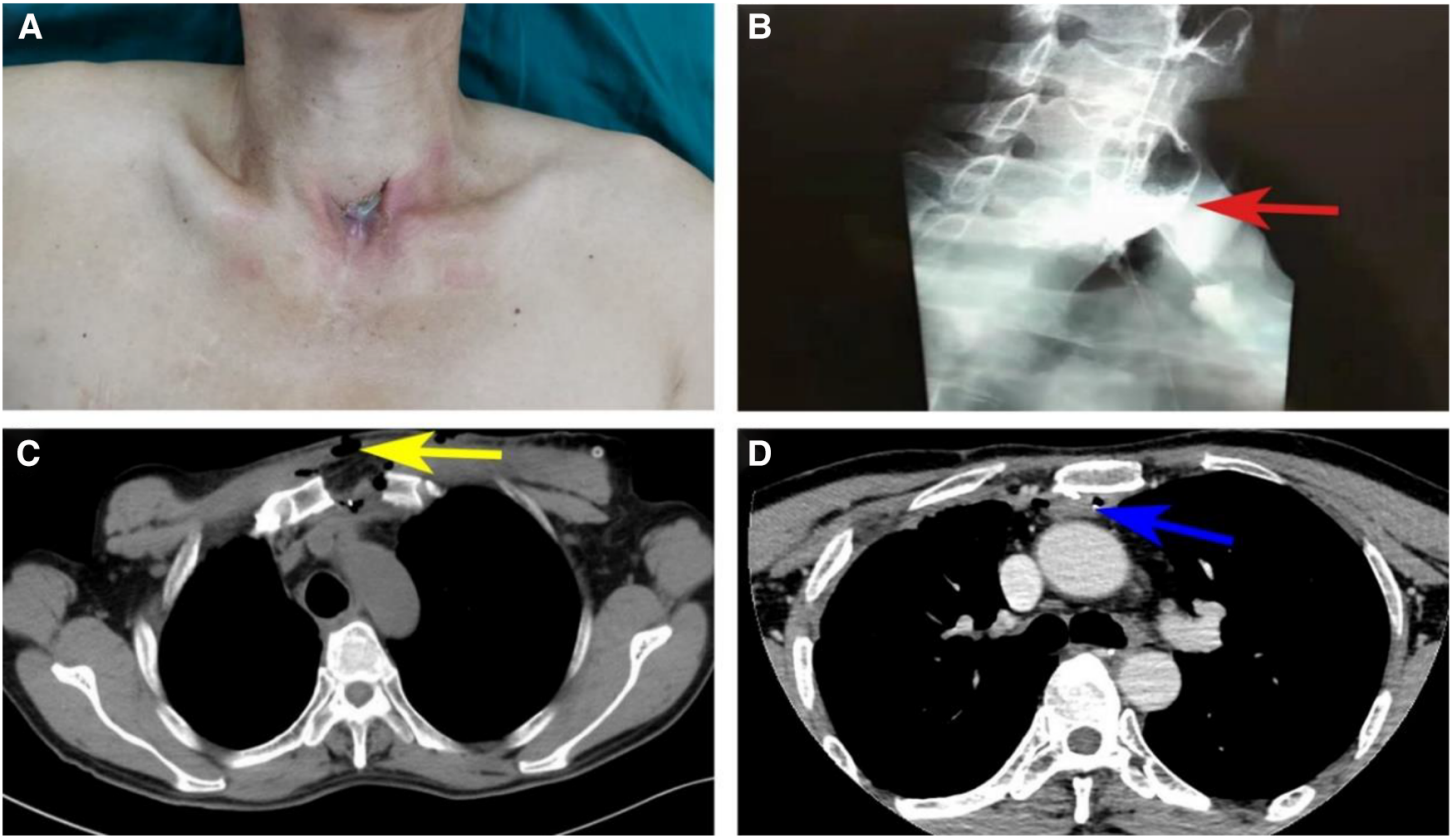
Preoperative visual inspection, esophagography and CT. (A) Visual inspection of external neck skin and esophageal cutaneous fistula; (B) Left lateral view from a barium swallow shows ingested barium extravasated from a perforation (red arrows) into extraluminal space with the lower part of the esophagus not visible, indicating esophageal stenosis and fistula. (C) CT shows the esophageal cutaneous fistula (yellow arrows). (D) CT shows the lower end of esophageal stricture (blue arrows) in T5.
